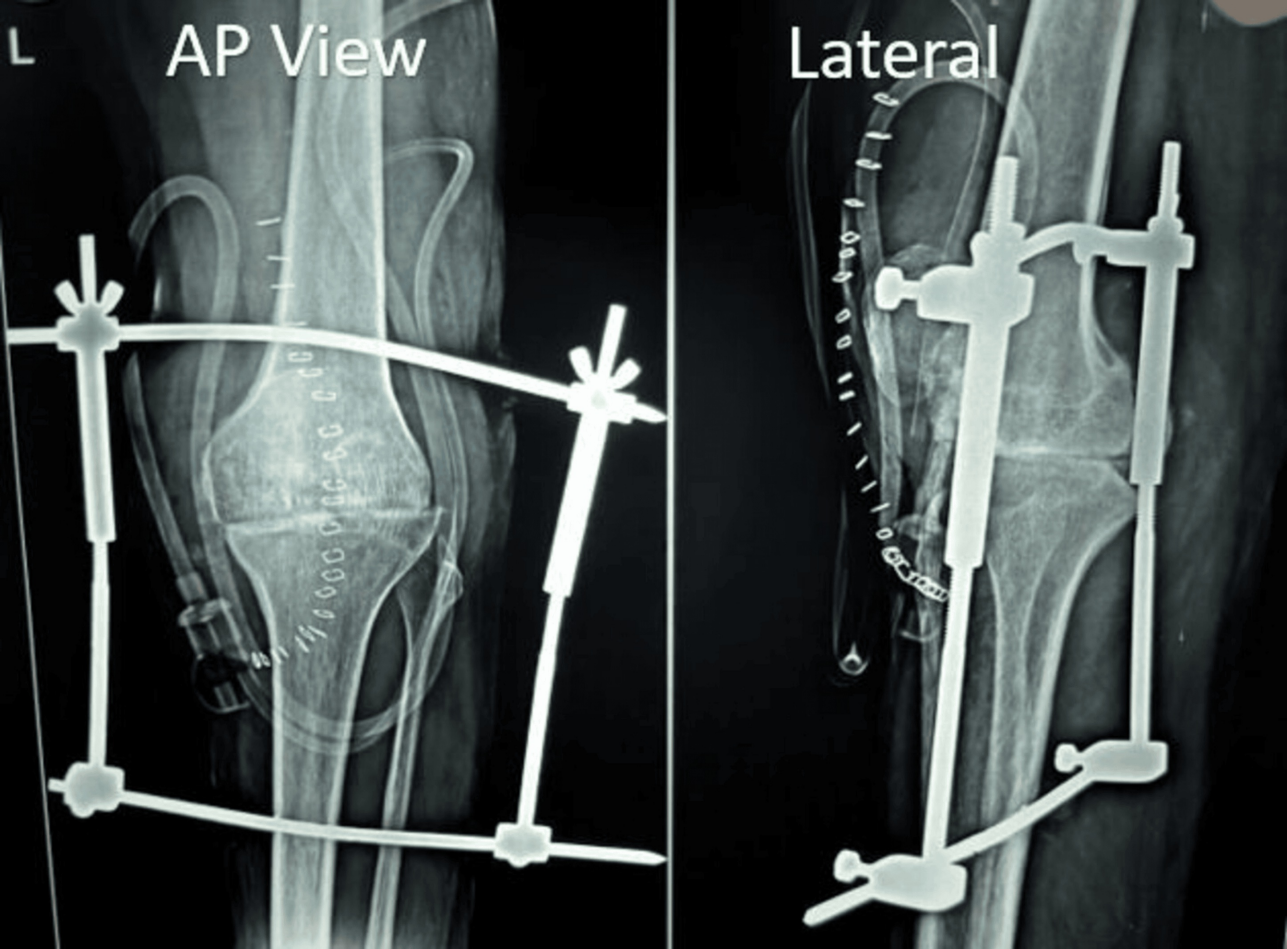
Immediate postoperative X-ray.
